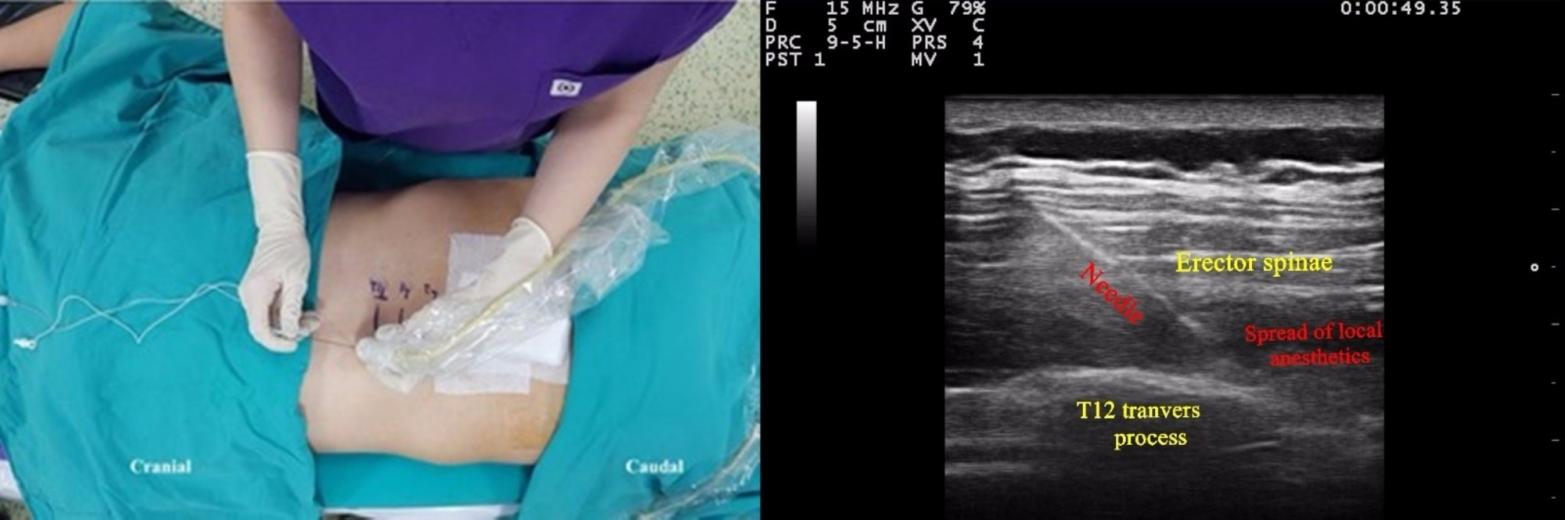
Patient position and sonographic anatomy of ESP ESP: erector spinae plane
